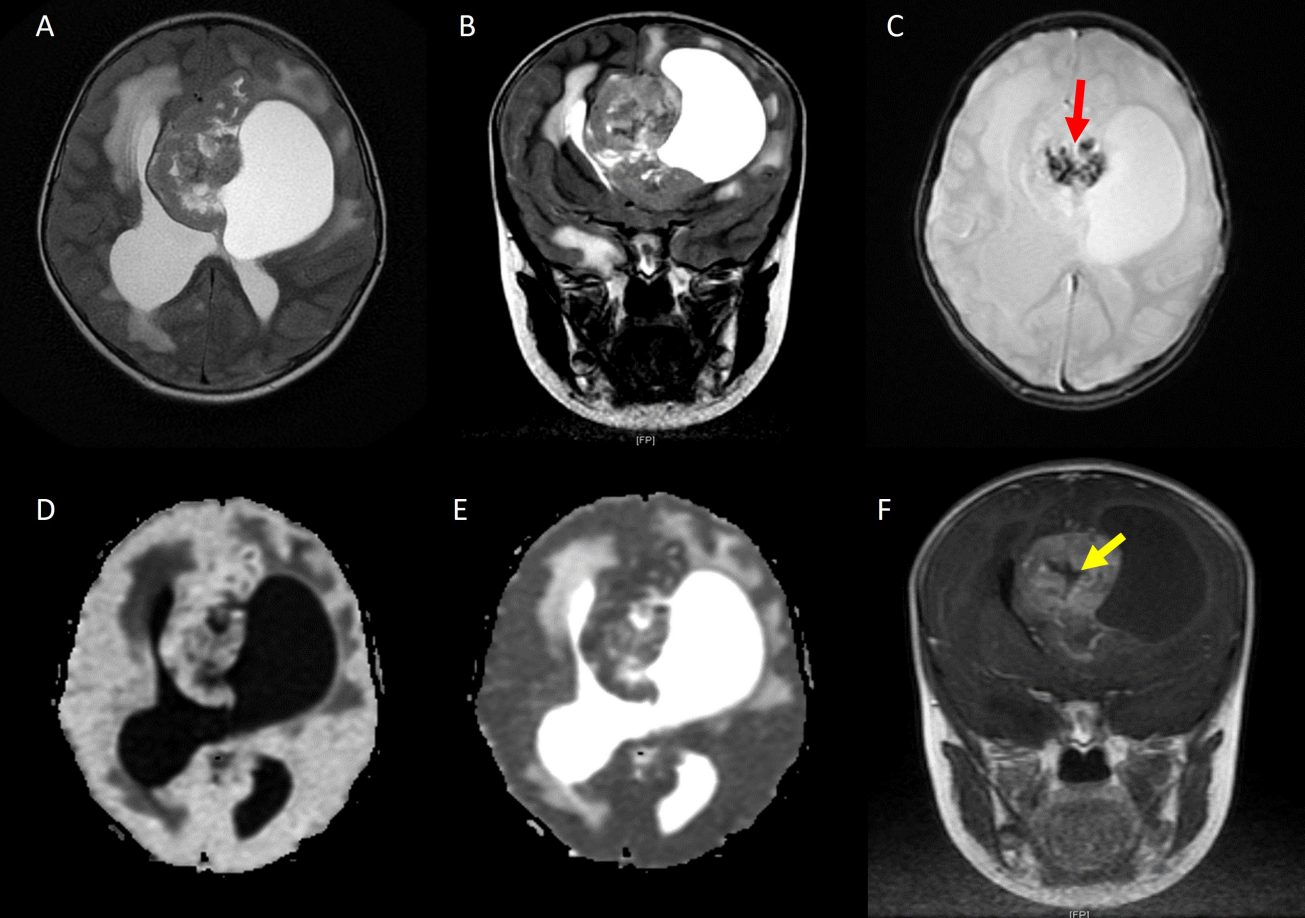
STE in periventricular location. A. Axial T2W image and B. coronal T2W image showing a heterogeneous solid cystic mass in the periventricular region; C. axial SWI showing foci of blooming (red arrow) suggestive of calcification/hemorrhage; D. diffusion-weighted imaging (DWI) shows the tumor is bright; E. apparent diffusion coefficient (ADC) shows hypointense signal suggesting low ADC value; F. coronal contrast-enhanced T1W image shows heterogeneous post contrast enhancement with the central non-enhancing area (yellow arrow) suggestive of necrosis
